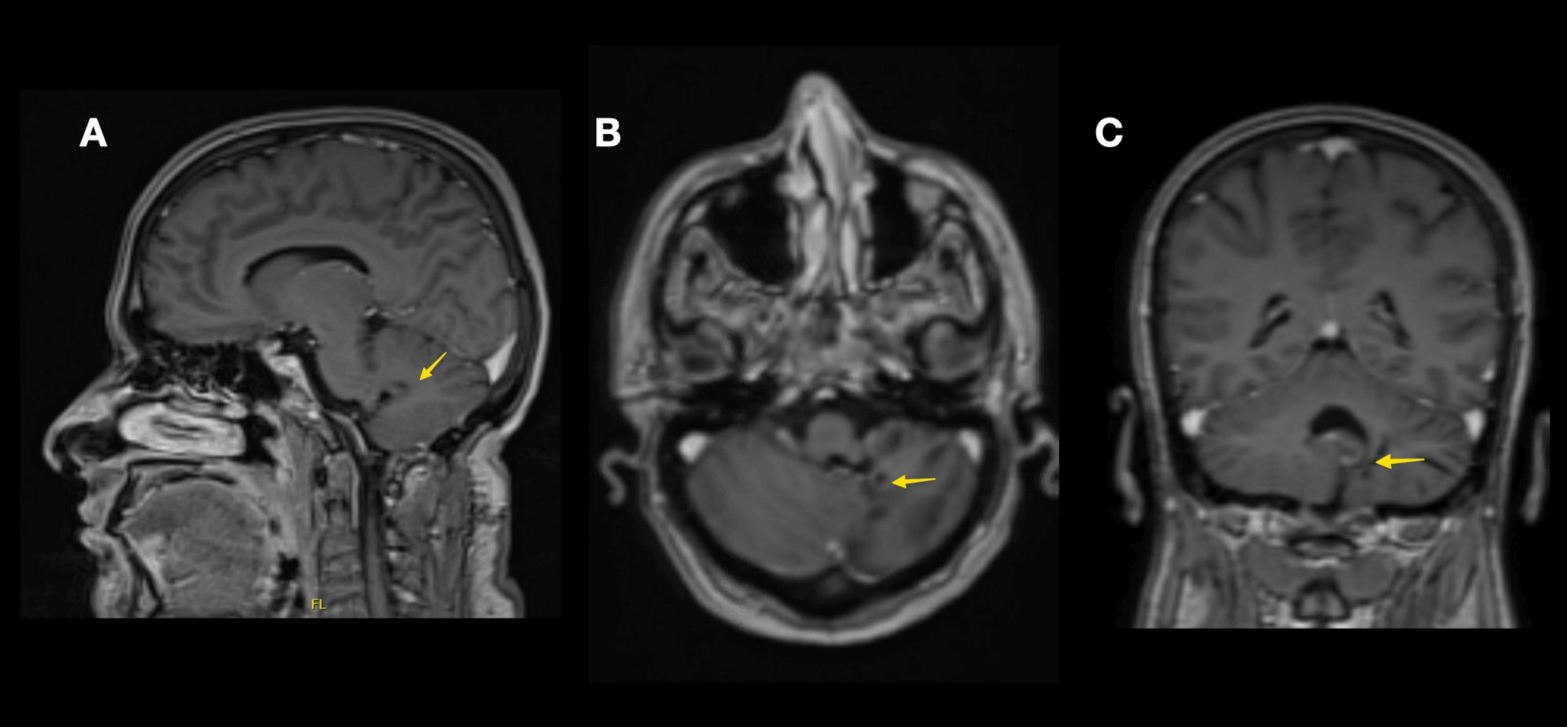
Brain MRI (with contrast) scan obtained after one year Brain MRI with contrast scan at one-year follow-up in sagittal (A), axial (B), and Coronal (C) views revealed interval resolution of previously noticed lesion of nodular enhancement (yellow arrow) within the left cerebellar hemisphere.
